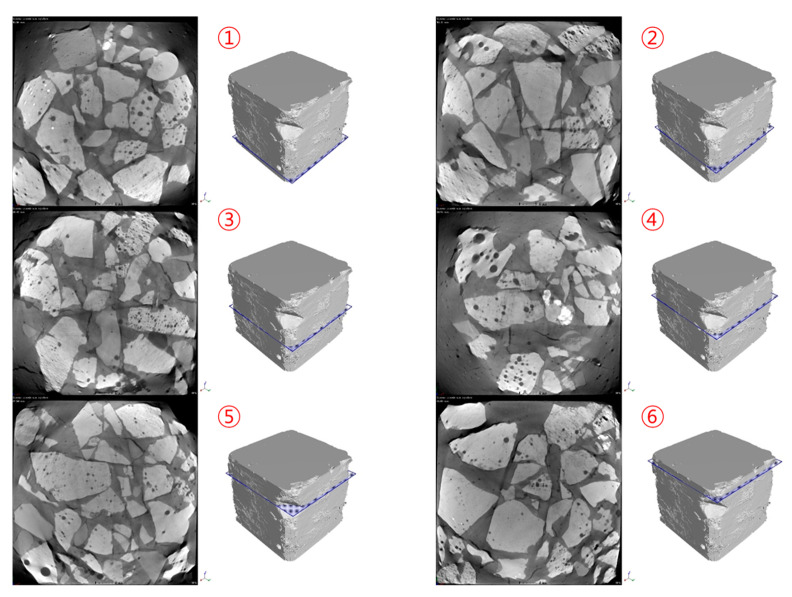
CT scanning process of mortar.
